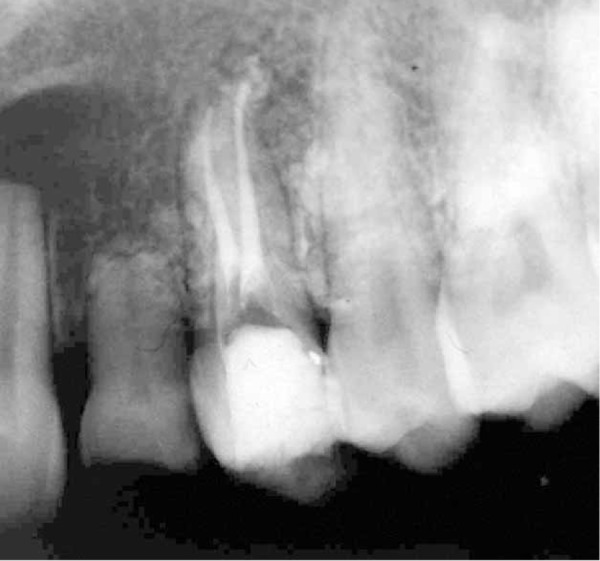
Radiograph showing complete root canal therapy
